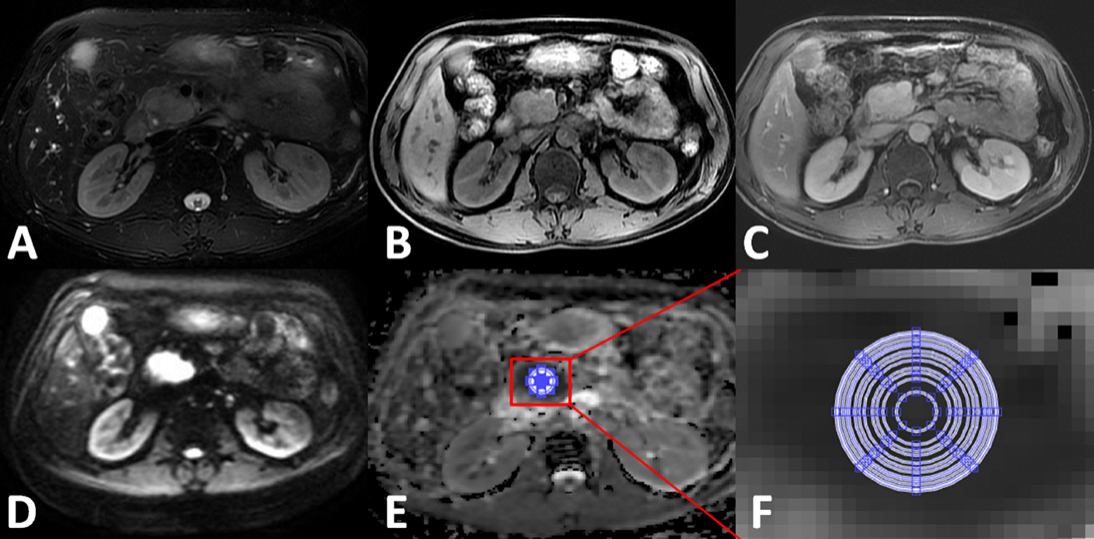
Apparent diffusion coefficient (ADC) measurements for mass-formed chronic pancreatitis at the head of the pancreas (A) Axial T2WI; (B) Axial precontrast T1WI; (C) Axial contrast-enhanced arterial phase T1WI; (D) DWI image (b = 600 s/mm^2^) clearly demarcated hyperintensity while compared with the surrounding pancreas tissues; (E) ADC map; (F) zoomed-in ADC map that indicates 12 concentric round ROIs (areas 15, 46, 55, 82, 99, 121, 134, 161, 189, 214, 223, and 245 mm^2^ were used for mean ADC measurements.
